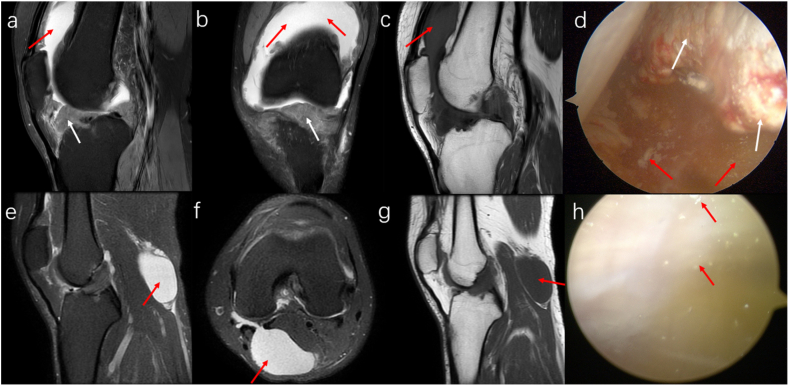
MRI and arthroscopic images of joint effusion, synovial proliferation, and popliteal cysts. A 33-year-old male with a 14-year history of KGA exhibits significant joint effusion in the suprapatellar bursa on sagittal (a) and coronal (b) PDWI-FS sequences, marked by strip-like high T2 signal intensity areas. These include diffusely low T2 signal intensity areas (red arrows), resembling the ultrasound's snowstorm sign. Sagittal T1WI sequence (c) shows slightly low T1 signal intensity (red arrows). Extensive synovial hyperplasia within the joint cavity is noted (white arrows), appearing as low T1 and moderate to slightly low T2 signal intensity (a–c). Arthroscopic examination (d) confirms synovial hyperplasia (white arrows) and intra-articular MSU crystal deposition (red arrows). Another patient, a 30-year-old male with a 4-year history of KGA (e–h), exhibits a popliteal cyst behind the knee on sagittal and axial PDWI-FS sequences (e, f; red arrow), showing high T2 fluid signal intensity. Low T2 signal intensity areas within the cyst are not clearly visible on the T1WI sequence (g). Arthroscopy (h) reveals MSU crystal deposition within the cyst (red arrow).
